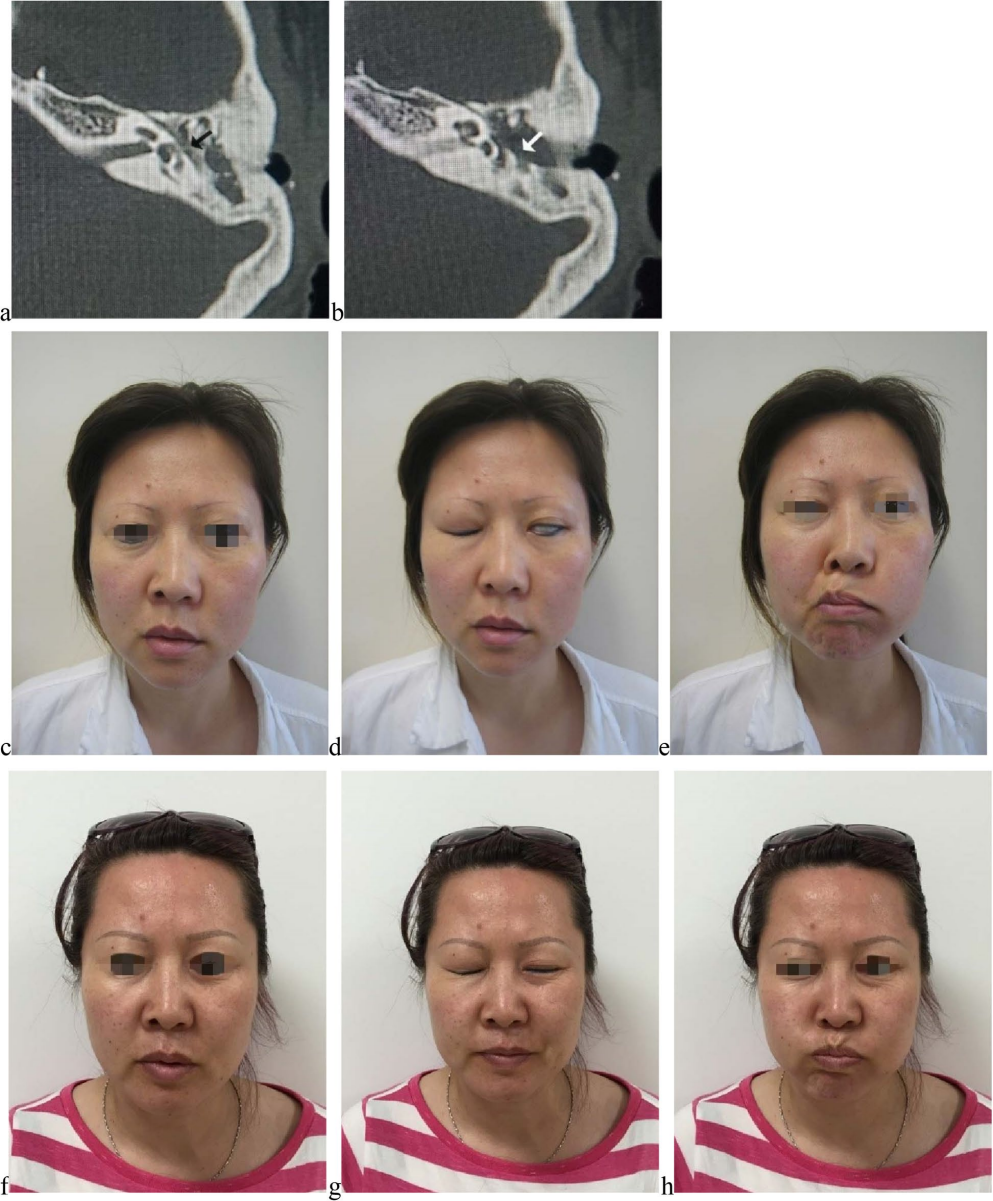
Recovery from H-B grade VI to H-B grade III after direct anastomosis. No. 18 patient, female, 37-year-old, suffered from more than 1/3 facial nerve transection in the canal wall down mastoidectomy for left-side cholesteatoma of middle ear, received direct anastomosis within 25 days. a b Axial CT images revealed fallopian canal of tympanic segment and the second genu were defective. c d e Facial images before graft; c at rest before anastomosis; d eye closure before anastomosis; e upper and lower lip movement before anastomosis. f g h Facial images after anastomosis; f at rest after anastomosis; g eye closure after anastomosis; h upper and lower lip movement after anastomosis
